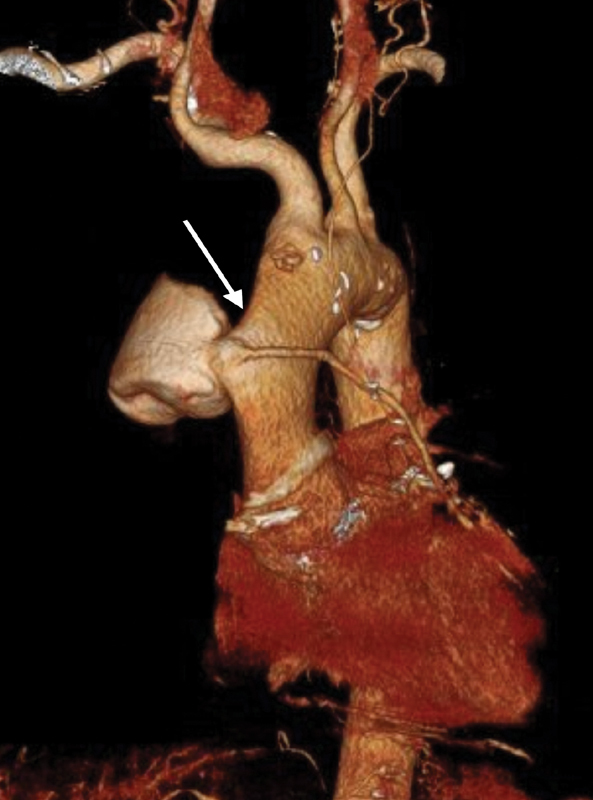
Preoperative computed tomography (CT) angiogram three-dimensional (3D) reconstruction; arrow indicates the anastomotic pseudoaneurysm and the patent venous graft.
